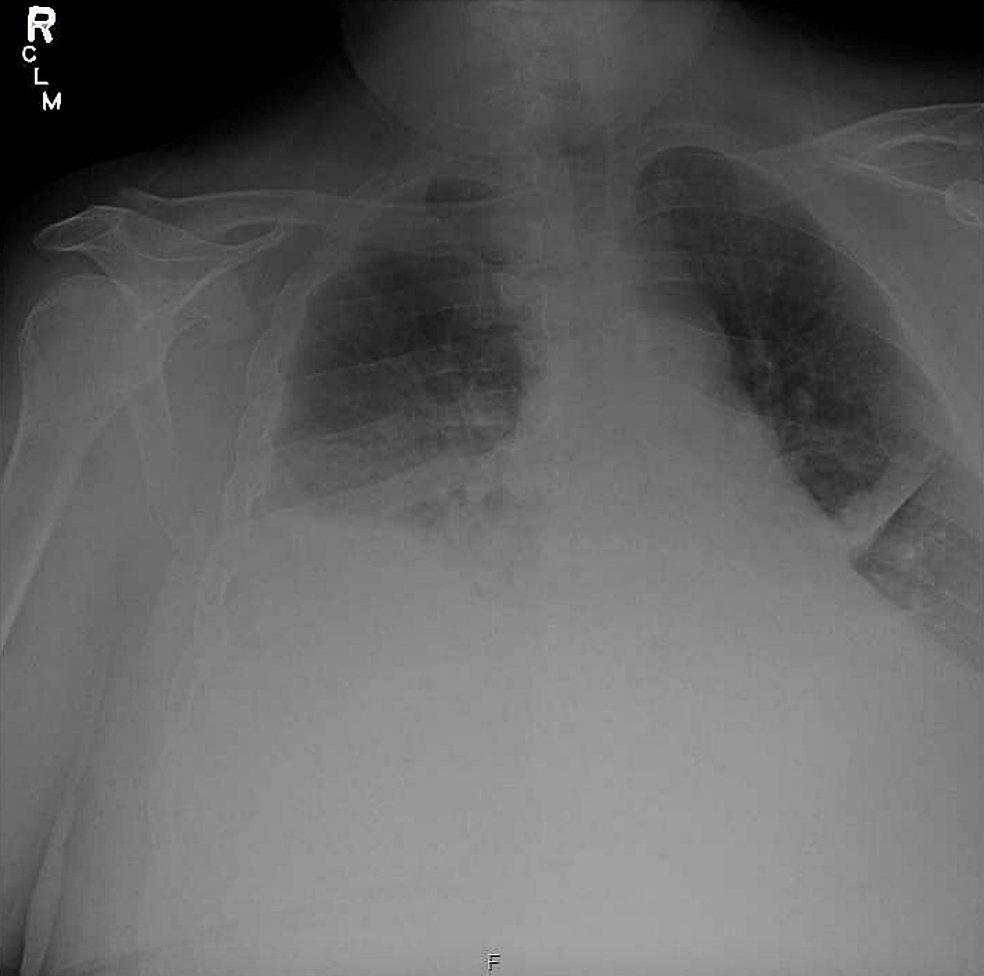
Chest radiograph showing bilateral pleural effusion
